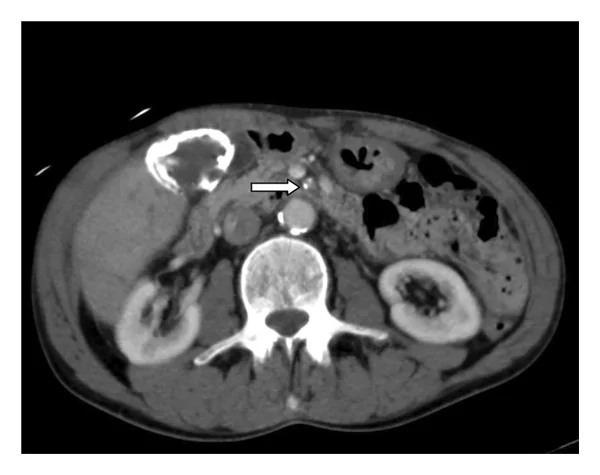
Contrast‐enhanced axial abdomen CT revealing narrowing of the middle segment of the SMA due to the presence of mixed plaque. (Arrow) The mixed plaque, containing both calcified and soft components, results in a reduction of the vessel lumen, potentially leading to compromised blood supply to the bowel, which could cause symptoms of ischemia.
